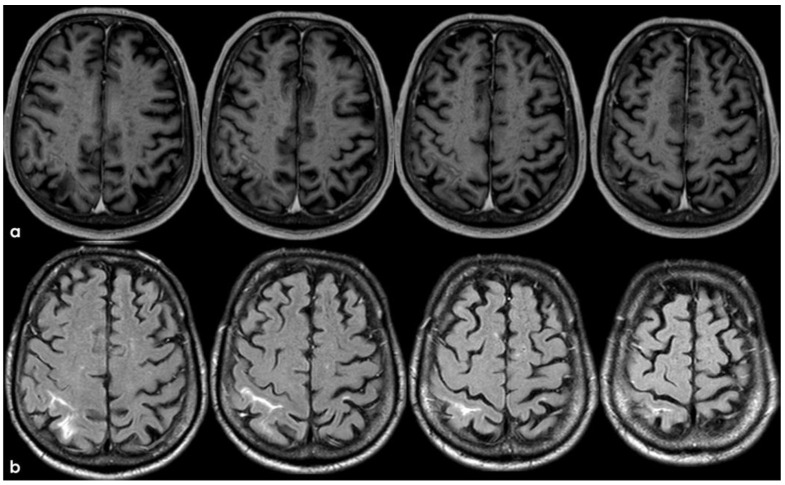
Brain MRI: post-contrast axial T1WI (panel (a)) and axial FLAIR (panel (b)), showing the contrast enhancement of the right post-central sulcus, corresponding with cSAH.
